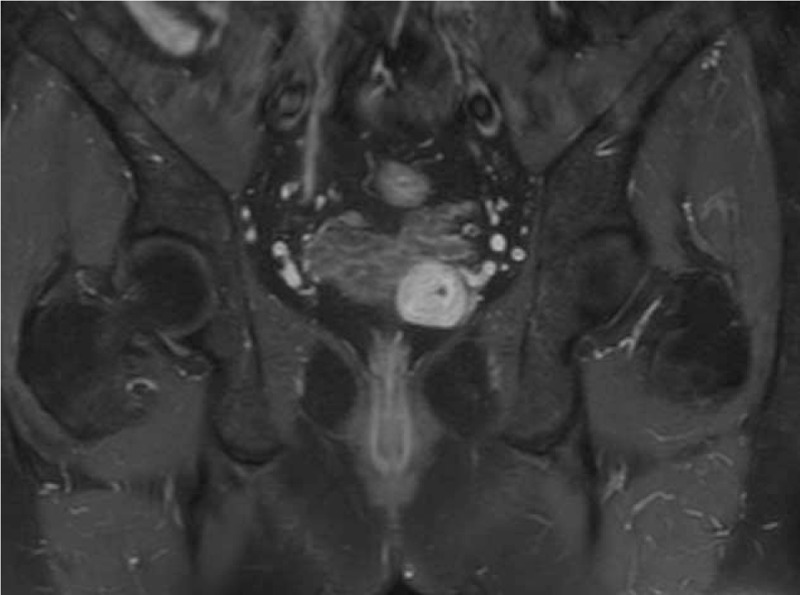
Pelvic MRI showed a solid enhancing mass on the left seminal vesicle on contrast-enhanced T1-weighted image.
